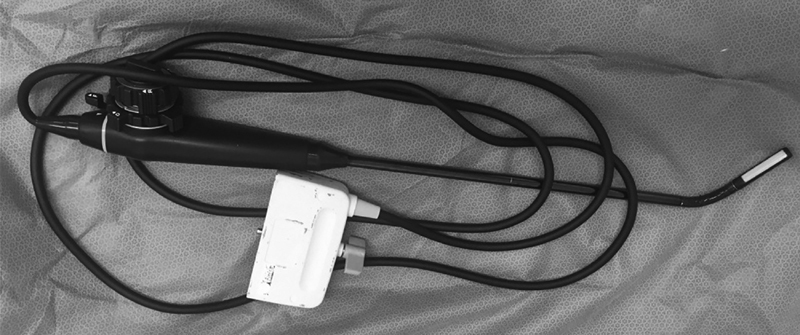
Ultrasound laparoscopic probe.
